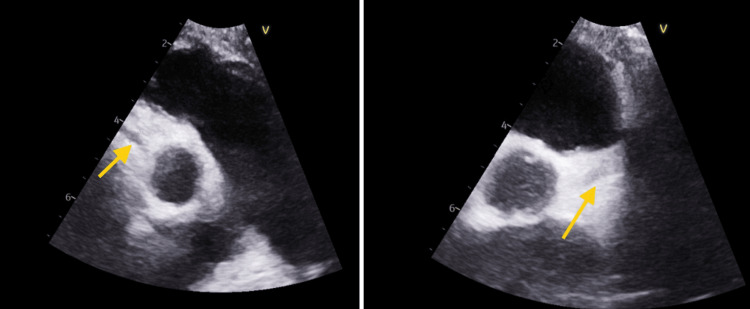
Echocardiography at presentation Left arrow: RCA 2.4 mm; Right arrow: LMCA 3.3 mm RCA: right coronary artery; LMCA: left main coronary artery
